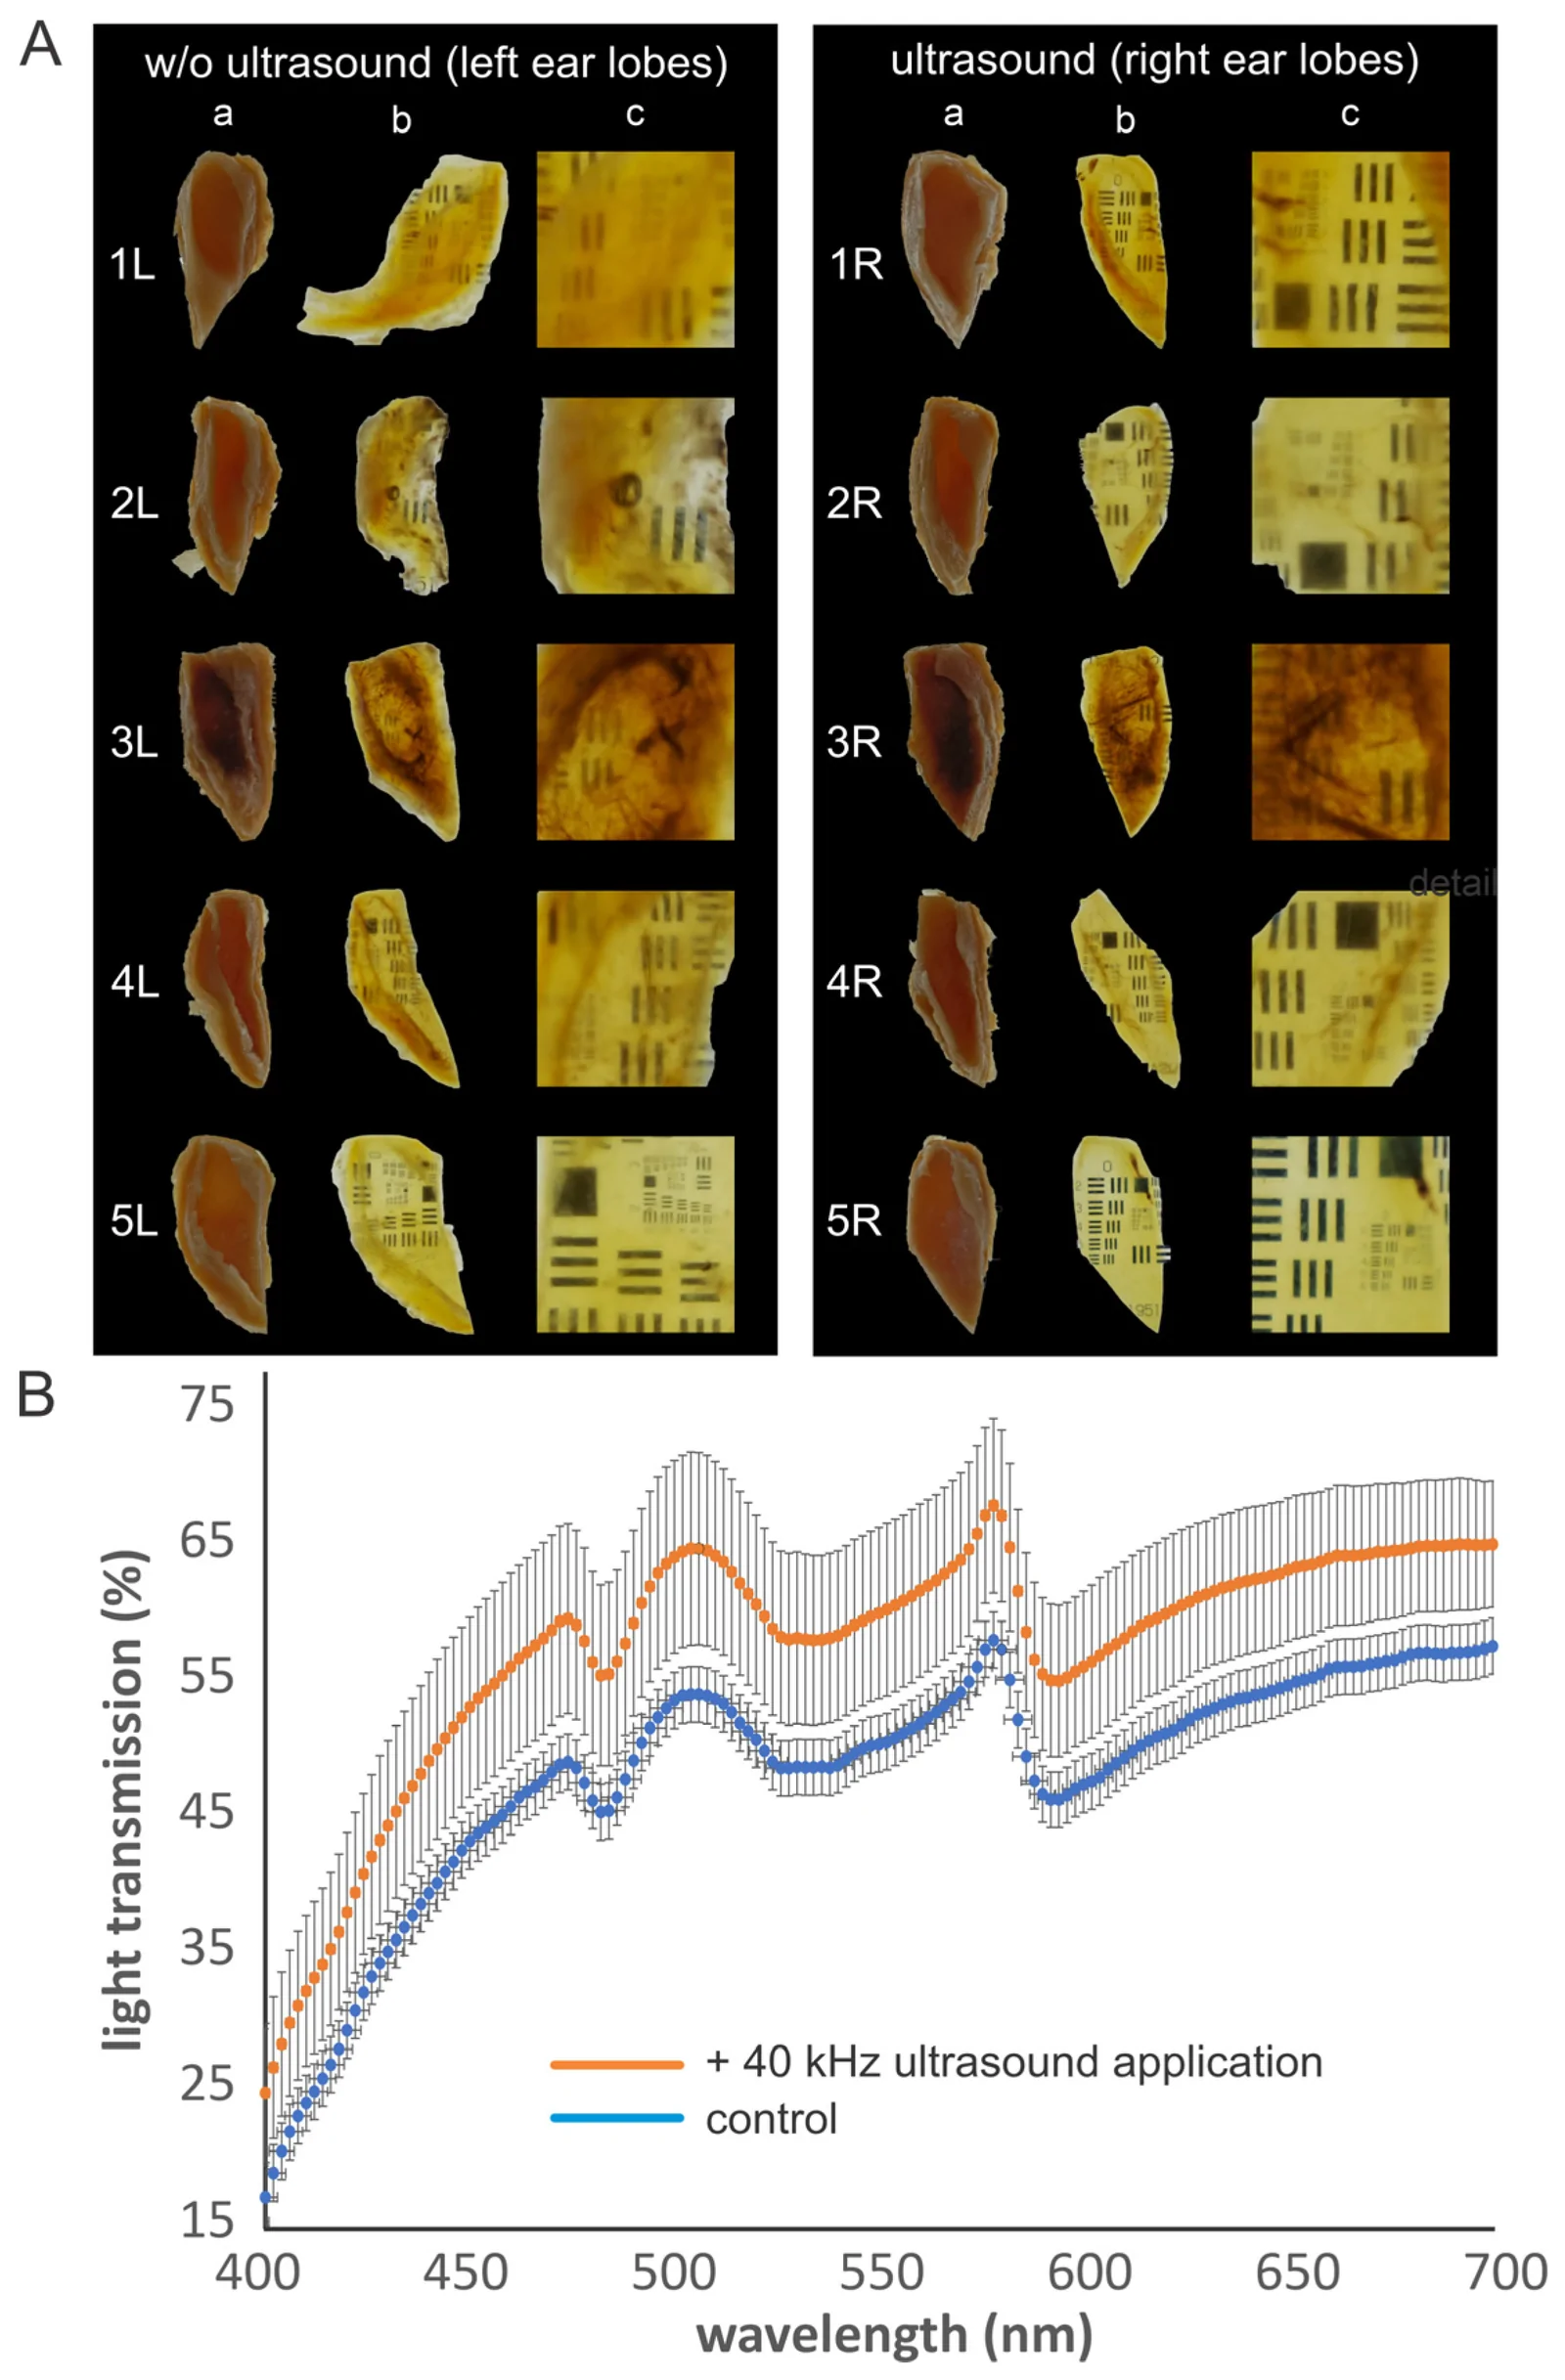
Long-term stability and quantification of transparency in human ear samples. (A) Comparison after one year of storage in DBE. The ultrasound-treated samples (right panel) remain visibly clearer than standard controls (left panel). (a) Uncleared samples. (b) Cleared samples. (c) Detail view of (b). For better assessment of transparency the samples were placed on an USAF test chart. (B) Transmission spectroscopy (400–700 nm) quantifies the improvement: ultrasound-treated samples (orange line) show significantly higher light transmission percentages compared to controls (blue line), confirming superior optical clarity even after one year of storage in DBE.
